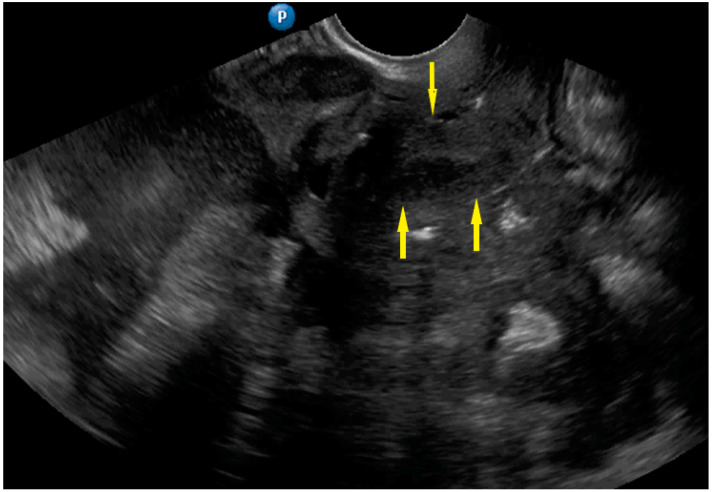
Ultrasound image of frozen pelvis. Yellow arrows identify uterus. There is involvement of bladder peritoneum and massive involvement of the compartment posterior to the uterus.
